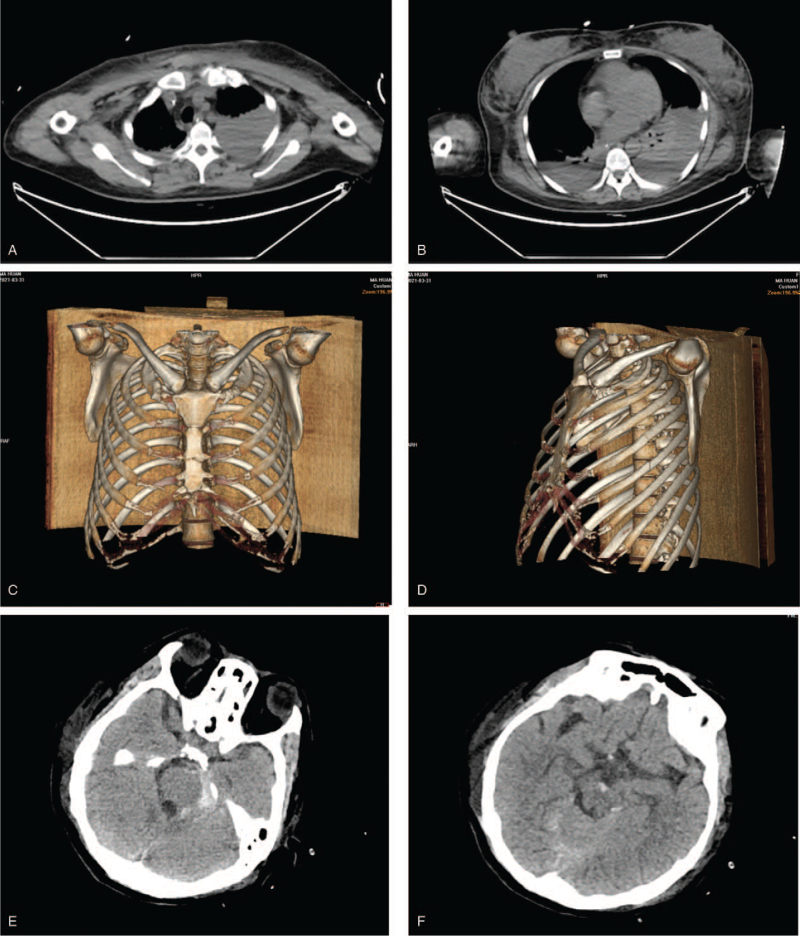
CT scans of the chest and brain in the ICU on day 1. (A) & (B) CT scans of the chest showed a large left pleural effusion. (C) & (D) CT scans of the chest showed fractures of the sternum and left ribs (4th and 5th ribs). (E) & (F) CT scans of brain showed subarachnoid hemorrhage. CT = computed tomography, ICU = intensive care unit.
